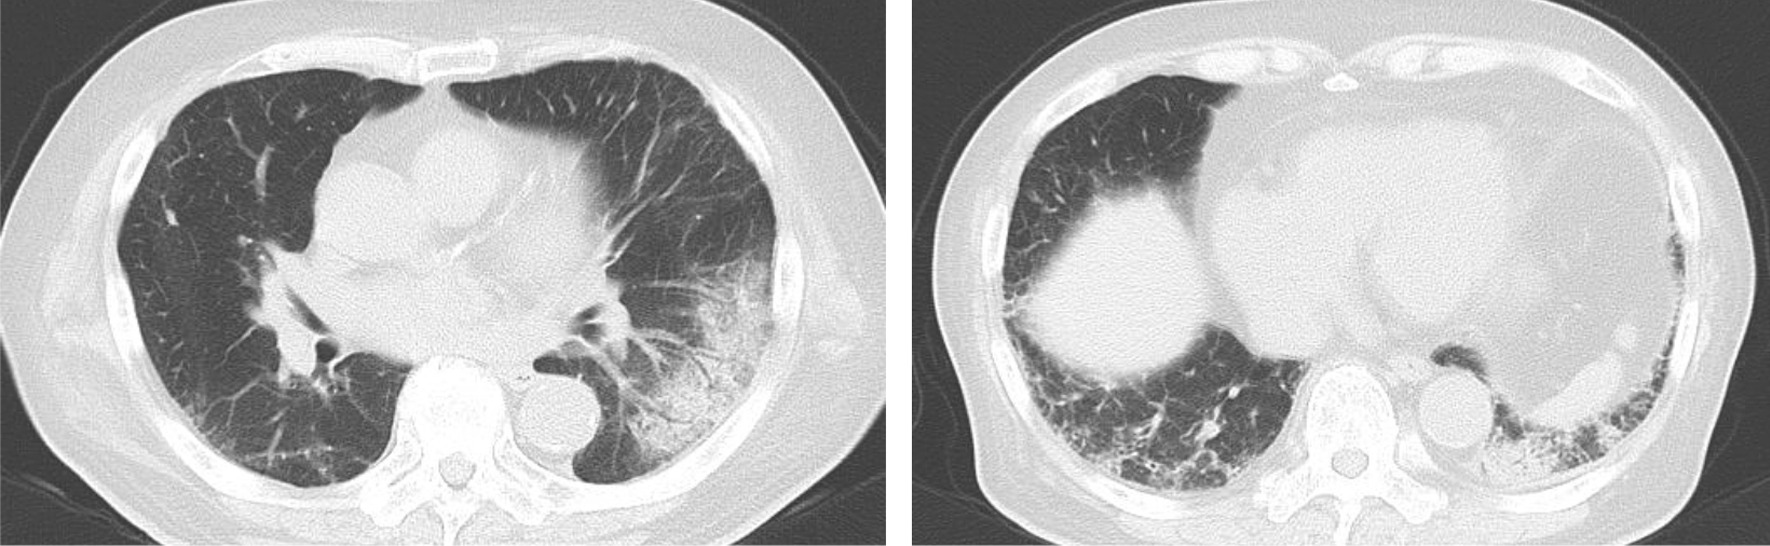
Chest CT at the first visit
